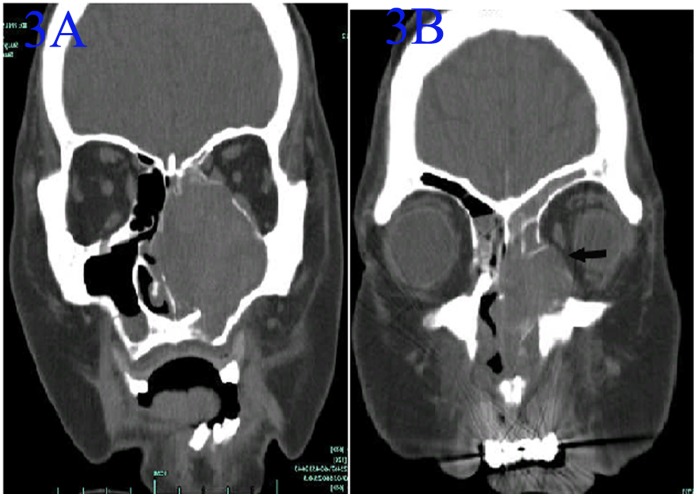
CT of an ANP in the left maxillary sinus involving the ipsilateral orbit. A :Noncontrast coronal CT in soft-tissue e window showed that a high-density mass filled the total left maxillary sinus. 3B: Noncontrast coronal CT in soft-tissue e window showed that the lesion was involved in the left orbitand the eyeball shifted laterally (arrow).
